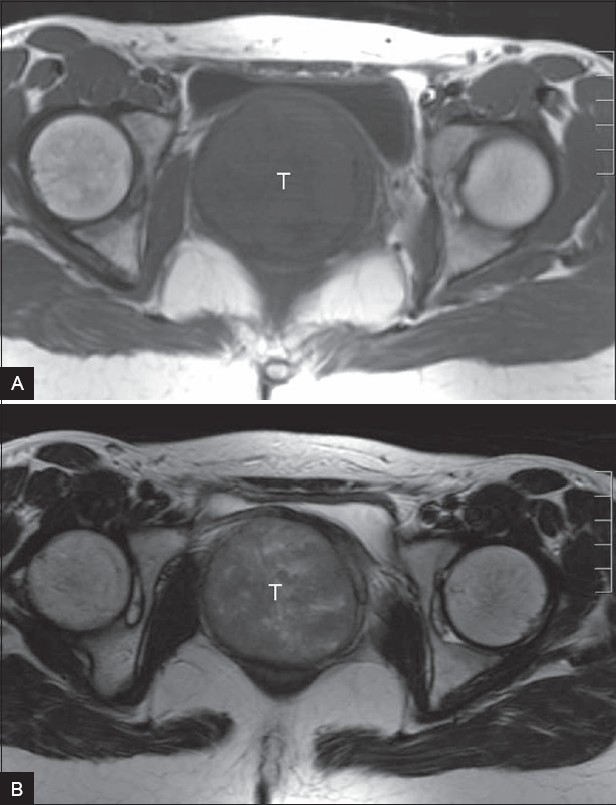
Axial T1W (TR / TE = 460 / 14) MRI (A) shows a wellcircumscribed, isointense, relatively heterogeneous, rounded (7.5 cm in diameter) pelvic mass (T) that displaces the urinary bladder anteriorly and compresses it. On the axial T2W (TR / TE = 5000 / 100) MRI (B), the mass (T) has an isointense signal with internal hyperintense irregular areas
